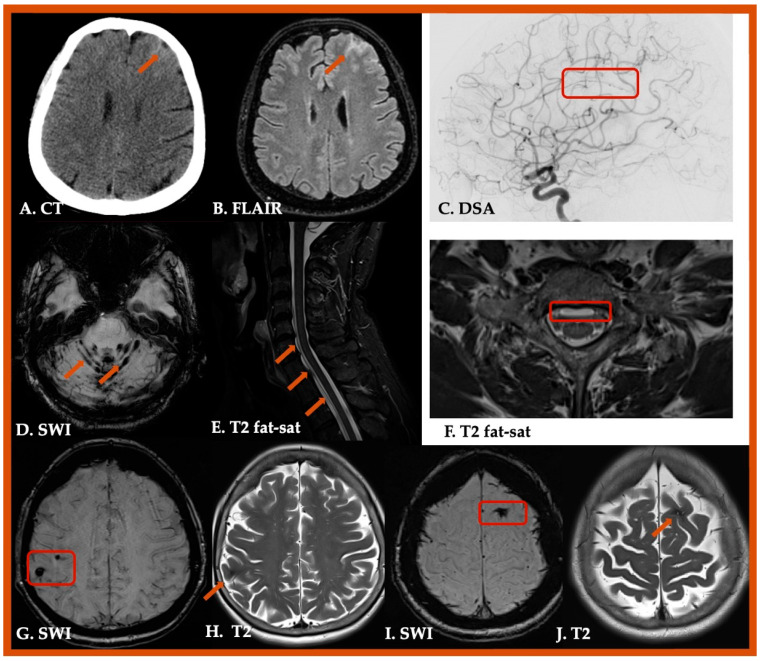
Radiological mimics of Cerebral Amyloid Angiopathy. A 68-year-old female patient with convexity subarachnoid hemorrhage, detected on brain Computed tomography (Panel (A)) and fluid-attenuated inversion recovery sequence (FLAIR) (Panel (B)), attributed to reversible cerebral vasoconstriction syndrome (RCVS) with characteristic vasoconstriction in the left pericallosal artery (Panel (C)). Another 58-year-old male patient with extensive infratentorial superficial siderosis (Panel (D), which was attributed to dural defect with extra-dural CSF, detected on T2-weighted fat saturated sequences. (Panels (E,F)). A 77-year-old female patient with multiple cortical and subcortical cerebral hemorrhagic lesions attributed to multiple cerebral cavernous malformations (Panels (G–J)).
